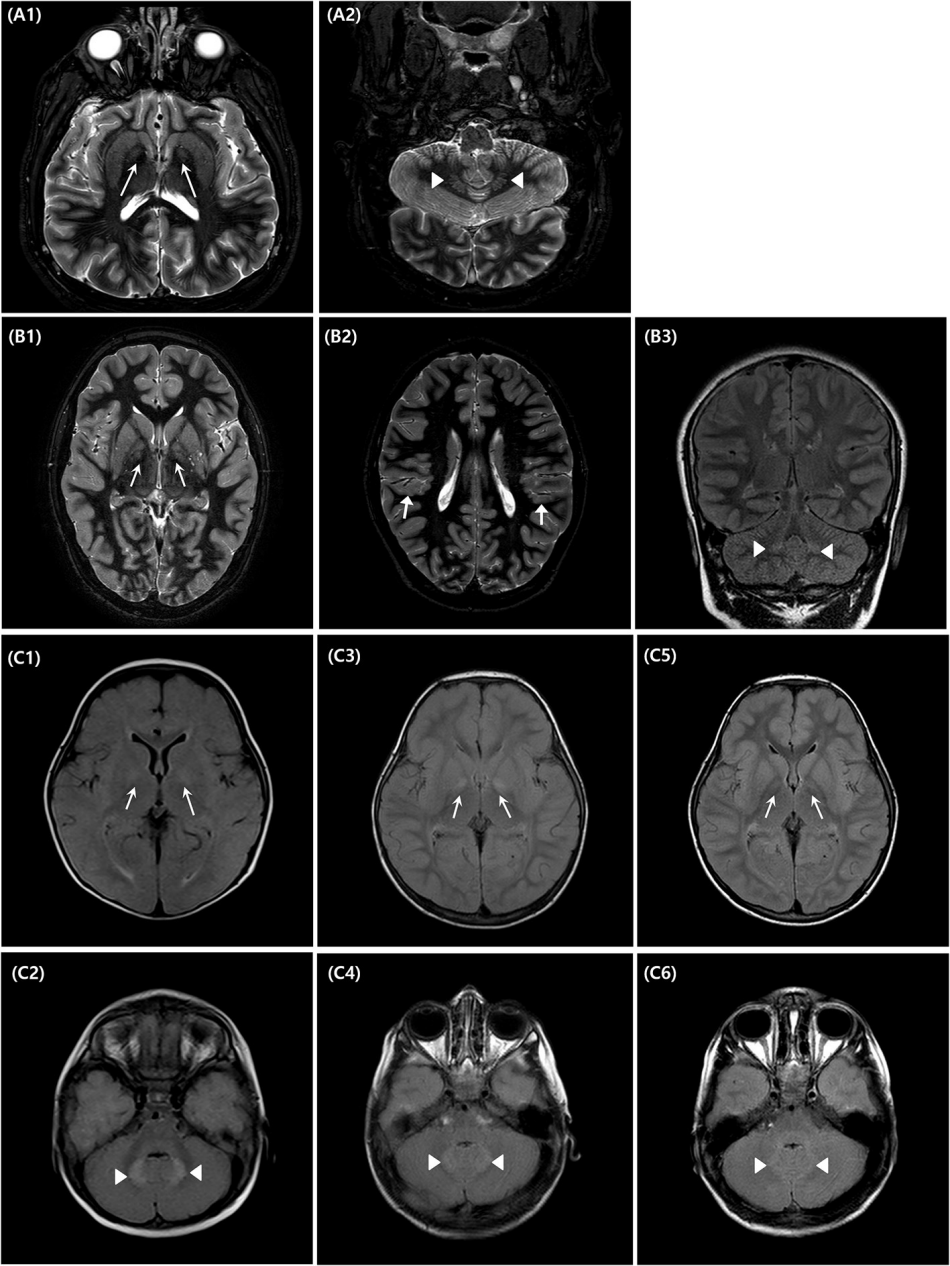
Brain magnetic resonance imaging of the affected individuals (IV-1, 4, 6). Three affected patients underwent brain magnetic resonance imaging (MRI) at different ages [(A)–Patient 1, (B)–Patient 2, (C)–Patient 3]. Patient 1 showed increased signal changes in the bilateral globus pallidi (A1) and the cerebellar dentate nuclei (A2) on T2-weighted axial images obtained at the ages of 22 years old. Patient 2 underwent brain MRI at the ages of 10 (B1–B3). There are bilateral high signal intensity lesions in the same area as patient 1, on T2-weighted axial and fluid-attenuated inversion recovery coronal images, and additionally, bilateral polymicrogyria around the perisylvian area are seen [(B2), arrow marks]. Patient 3 underwent a series of three brain MRIs when 8 months (C1,C2), 2 years (C3,C4), and 4 years old (C5,C6), respectively. Brain MRI performed at 2 years of age, showed the most distinct high signal intensity of bilateral globus pallidi (C3) and cerebellar dentate nuclei (C4).
